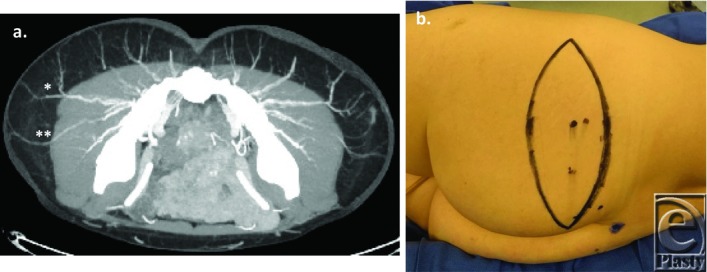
(*a*) CTA images were obtained at 2-mm intervals, along with 3-view thick slab maximum intensity projection images at 30-mm intervals. * indicates the dominant perforator.  indicates the secondary, lateral perforator. (*b*) Vessel locations were confirmed and marked intraoperatively using a pencil Doppler probe. An elliptical-shaped skin island was designed so that the perforators were centrally placed.
